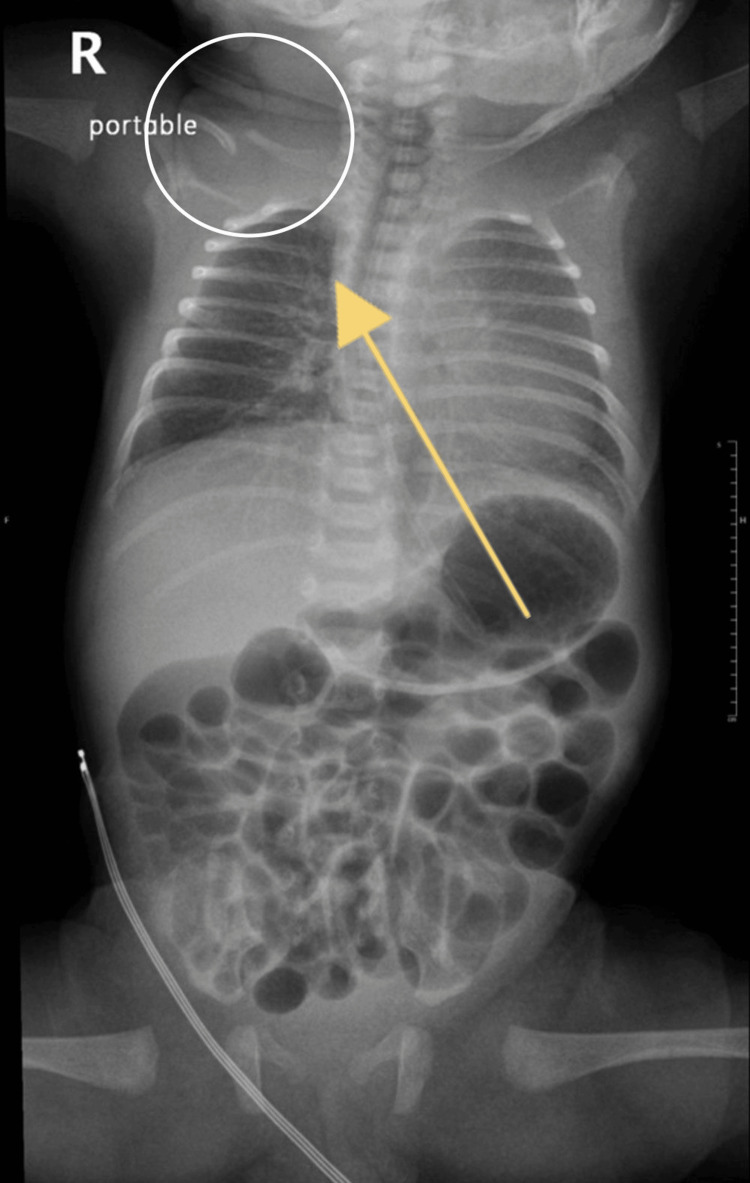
Chest X-ray of the second case showing the deformity of the clavicle on the right side.
